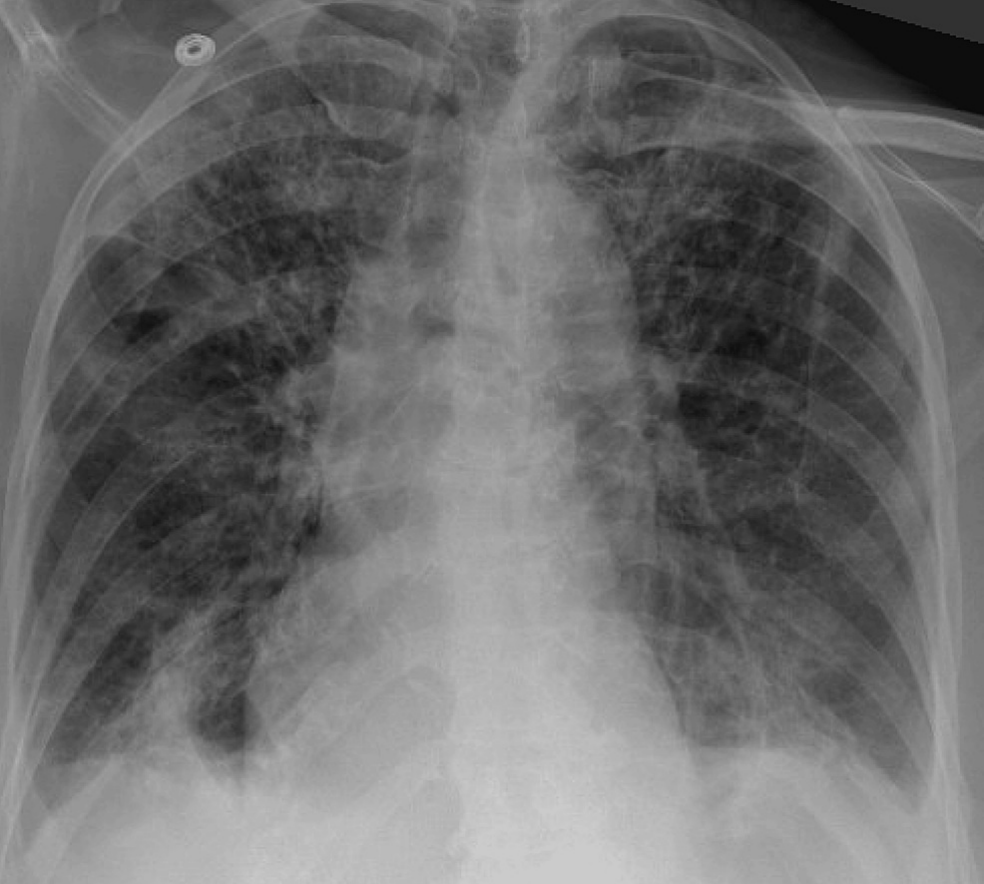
Chest X-ray six days after stopping daptomycin
